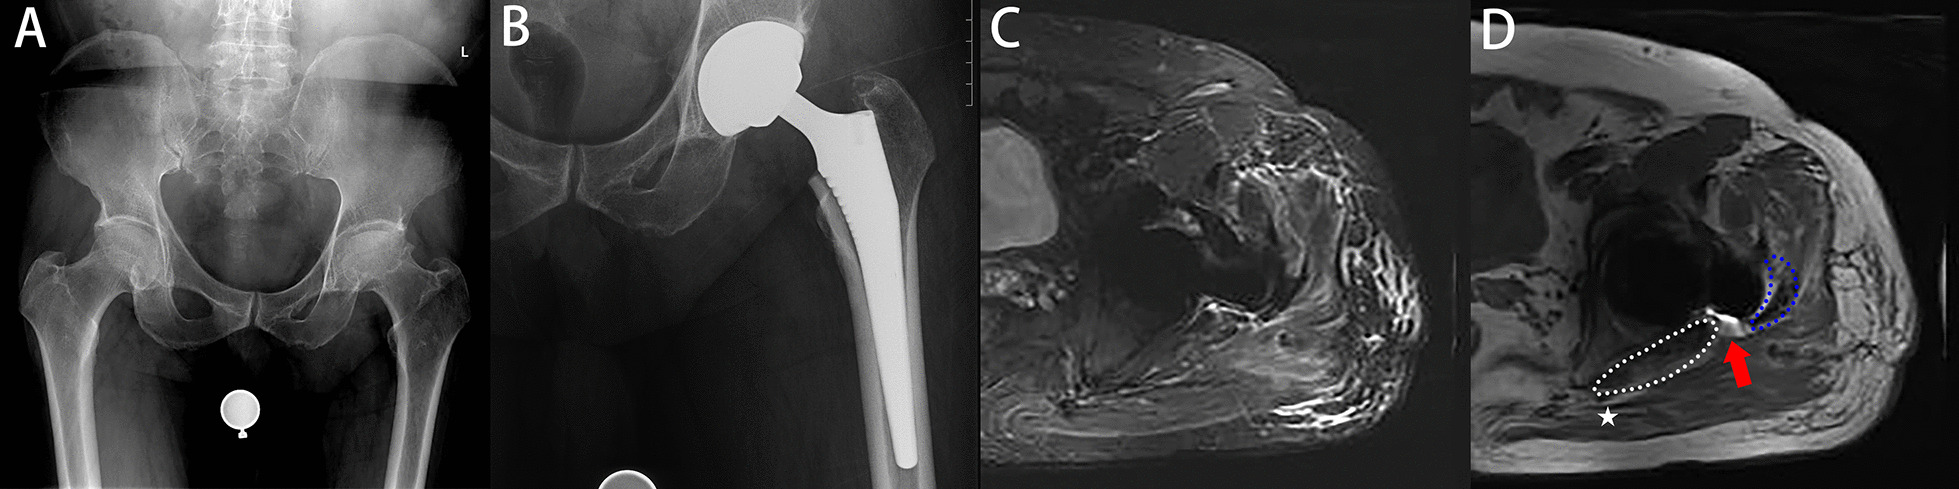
Male, 63 years old, osteonecrosis of femoral head (A). THA with conventional capsular repair was performed (B). Follow-up MRI (C, D) showed a gap between capsule and femur (red arrows). Symbols are identified as above
